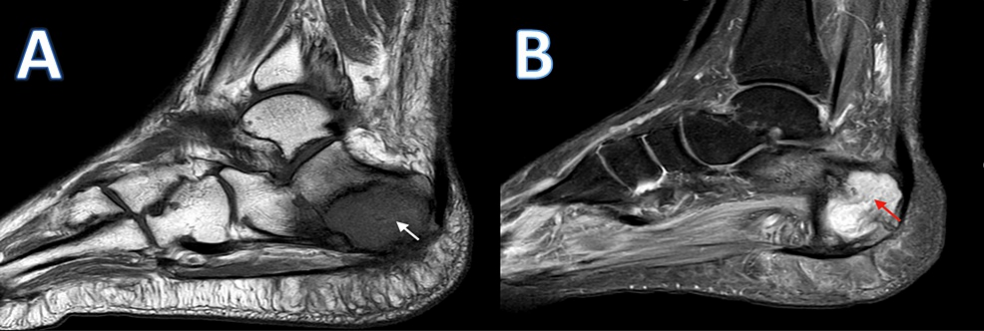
T1-weighted and T2-weighted MRI of the right foot (A) Right hypointense calcaneal marrow infiltrative lesion measuring 4.6x3 cm on T1 (white arrow) and (B) isointense to hyperintense on T2 (red arrow). The lesion demonstrates vivid enhancement after contrast administration, along with bone expansion and cortical breaching.
